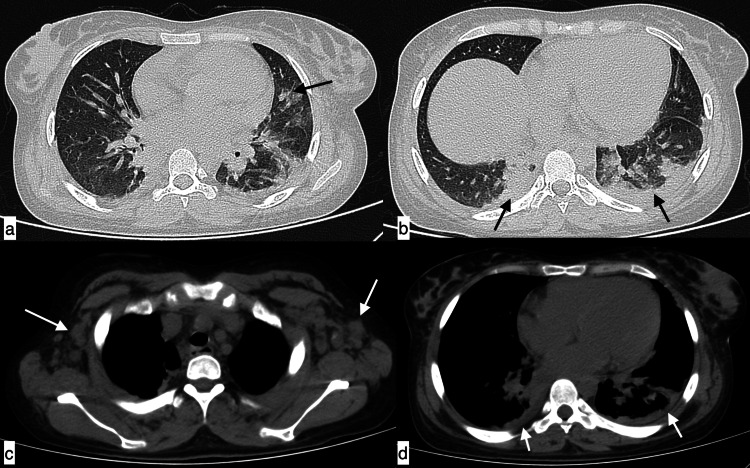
Lupus pneumonitis An 18-year-old female patient with SLE presented with fever, shortness of breath, skin rash, joint pains, and myalgias of five-day duration. Axial HRCT chest images in the lung window revealed patchy areas of consolidation (black arrows) in the lingular segment of the left upper lobe (a) and basal segments of bilateral lower lobes (b). Mediastinal window images showed enlarged bilateral axillary lymph nodes (c) and cardiomegaly with bilateral minimal pleural effusions (white arrows) (d). Imaging differentials included infection and lupus pneumonitis. The final diagnosis was lupus pneumonitis. SLE: systemic lupus erythematosus; HRCT: high-resolution CT
